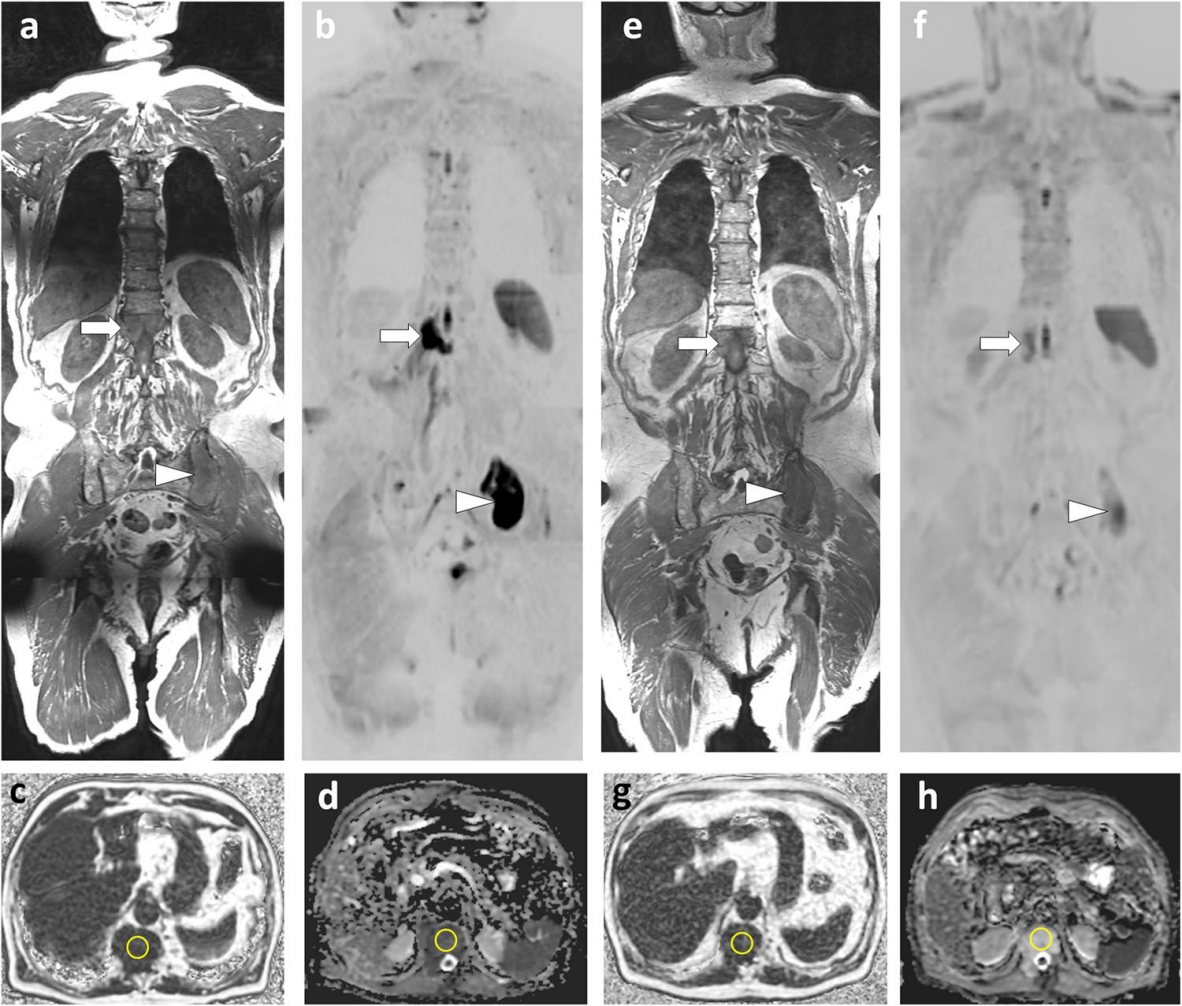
77-year-old man with multiple myeloma: WB-MRI studies at diagnosis and after treatment. a, b Coronal T1 (a) and high *b*-value (inverted grayscale window, *b* = 1000 s/mm^2^) DWI (b) sequences show a large lesion within the T12 vertebral body extending into the right paraspinal soft tissues (arrow). Another lesion is seen within the left posterior iliac crest (arrowhead). c Axial fat fraction (FF) map; measurement within the T12 body (circle) shows the absence of residual marrow fat (FF = 0%). d Axial ADC map; measurement within the T12 body (circle) shows increased ADC (750 mm^2^/s), indicating tumor infiltration. e–h Morphologic images and corresponding quantitative maps from the MRI follow-up examination performed two months later, after initiation of systemic treatment and irradiation of the lesions: regression in size and fading of the lesions on morphologic images (arrows and arrowheads in e, f). Measurements within the body of T12 show increased FF (16%) and ADC (1235 mm^2^/s) (circles), indicating lesion response to treatment
